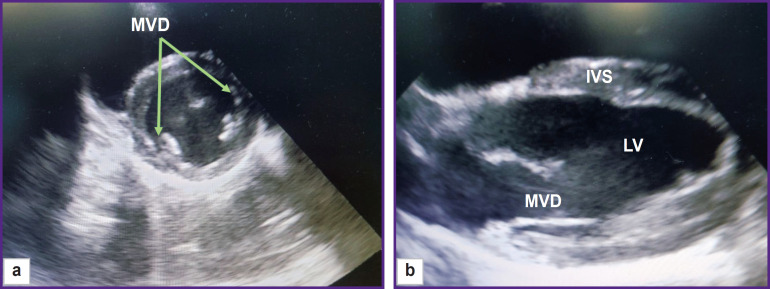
EchoCG image of the mitral valve; right parasternal projection: (a) short-axis view; (b) long-axis view; MVD — mitral valve diameter, IVS — interventricular septum, LV — left ventricle
